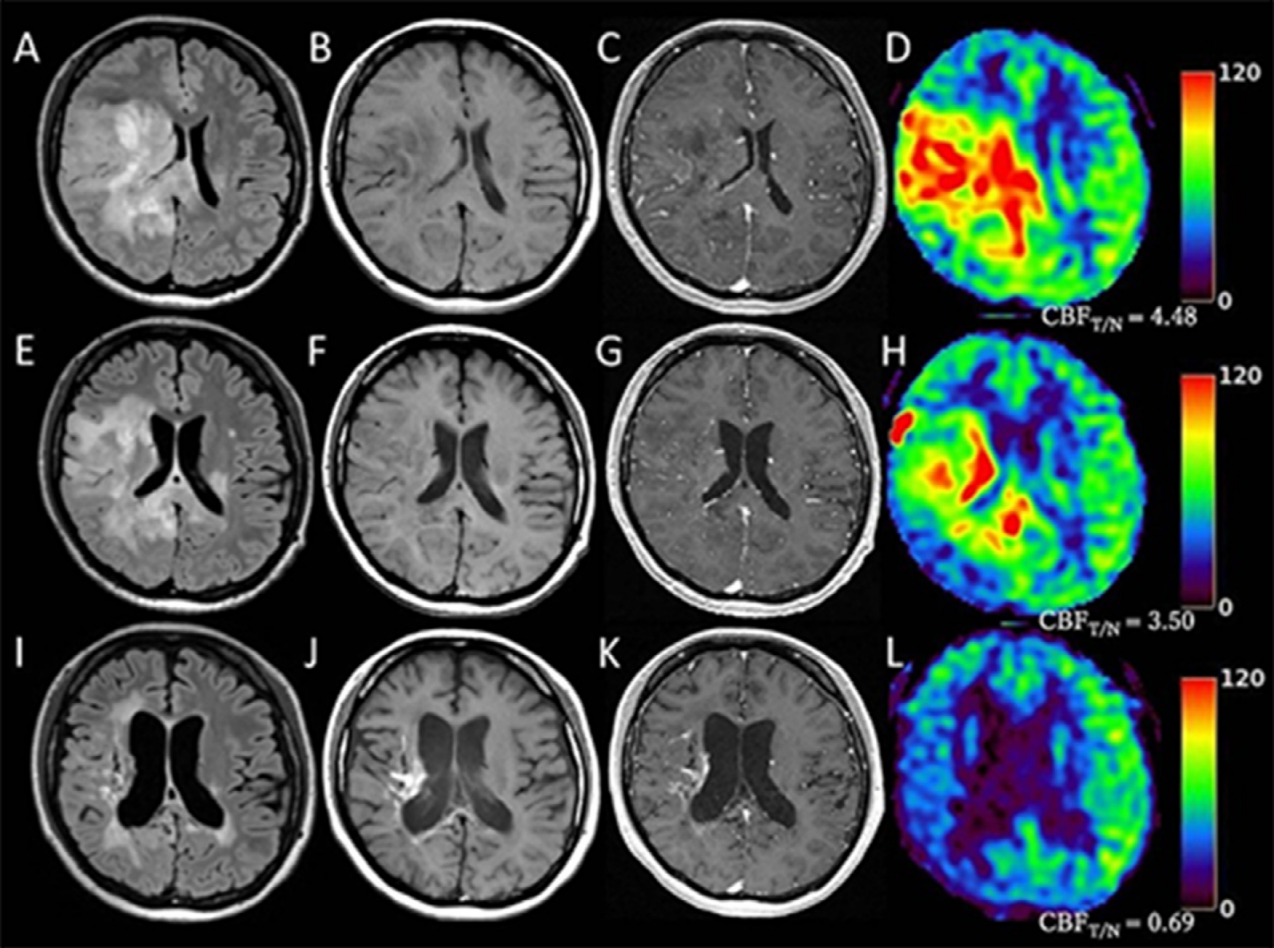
Imaging during treatment. (A-D) At the start of radiochemotherapy; (E-H) Two months after starting treatment; (I-L) 15 months after starting treatment. FLAIR (A, E, I), T1WI (B, F, J), Gd-T1WI (C, G, K), ASL (D, H, L).
